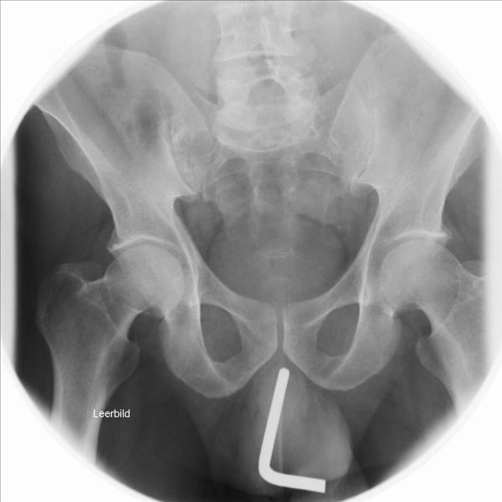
X-ray of the pelvis showing the Allen key in the urethra.
